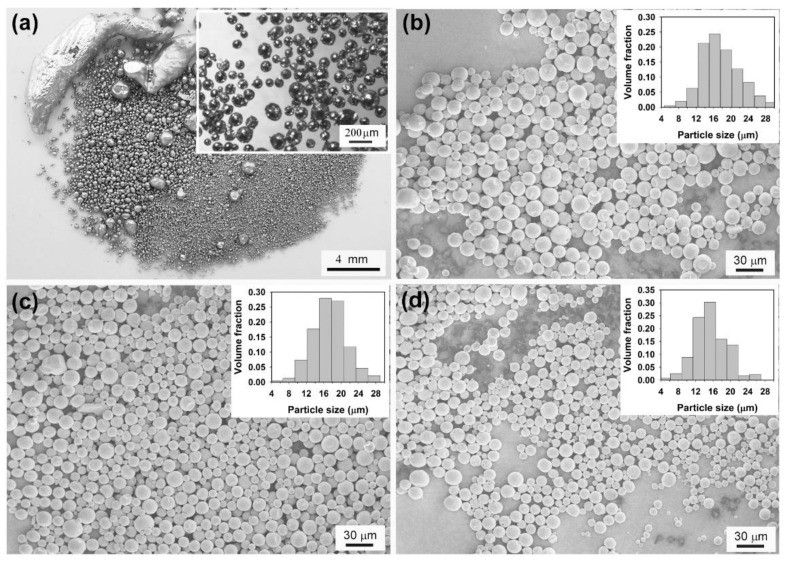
Optical or SEM images of the products obtained under different volume ratios of salts to metal: (a) 5:1, (b) 25:1, (c) 100:1, and (d) 200:1. Other experimental conditions: ultrasonic power = 1080 W and sonication time = 5 min. Inset is the optical image of Sn particles of small size present in the product or the volume-based particle size distribution.
